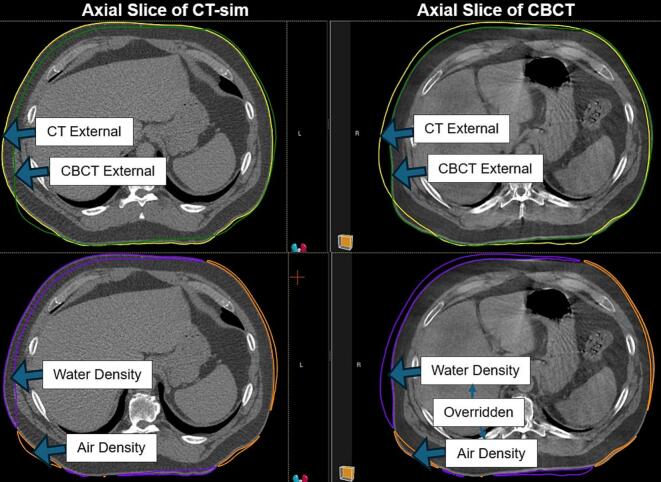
Images from the treatment planning system showing external and material override contours on CT-sim and HSTB-CBCT to account for surface changes.
